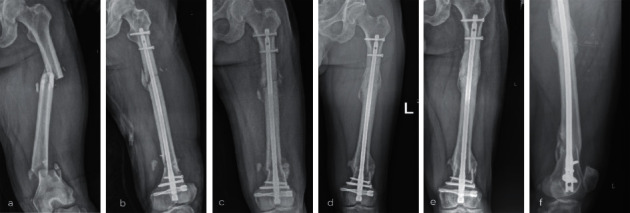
Male, 33, multi-fragment fracture of left femur with AO classification B3 in shaft, and A2 in distal part (a). Treated with retrograde nailing and postoperative MDI, 1-day postoperative radiograph (b). 1 month (c), 3-month (d) follow-up radiographs. 1-year follow-up radiographs indicated union (e, f).
